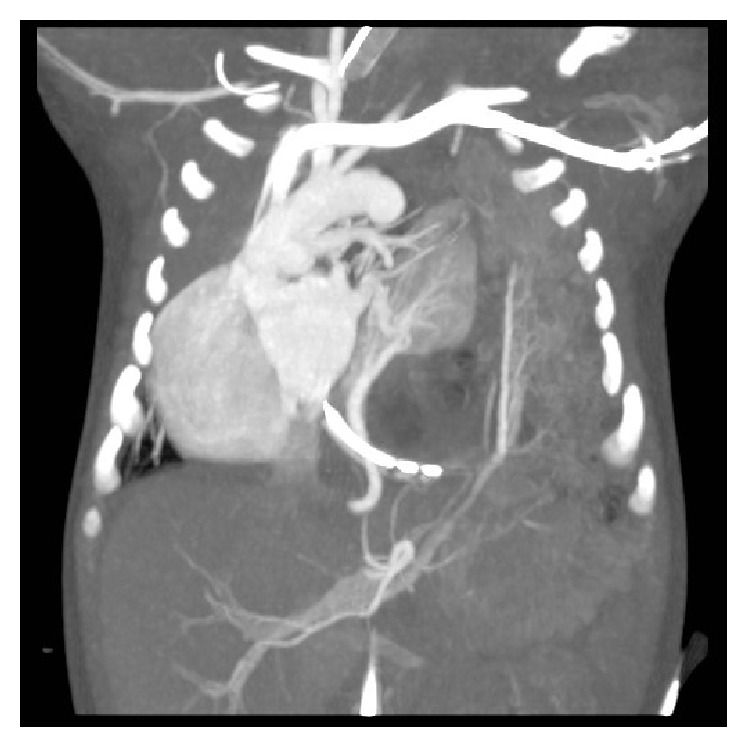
Coronal CT image showing feeding artery to sequestrated lung segment arising from the descending aorta and draining vein from the sequestration entering into left atrium.
